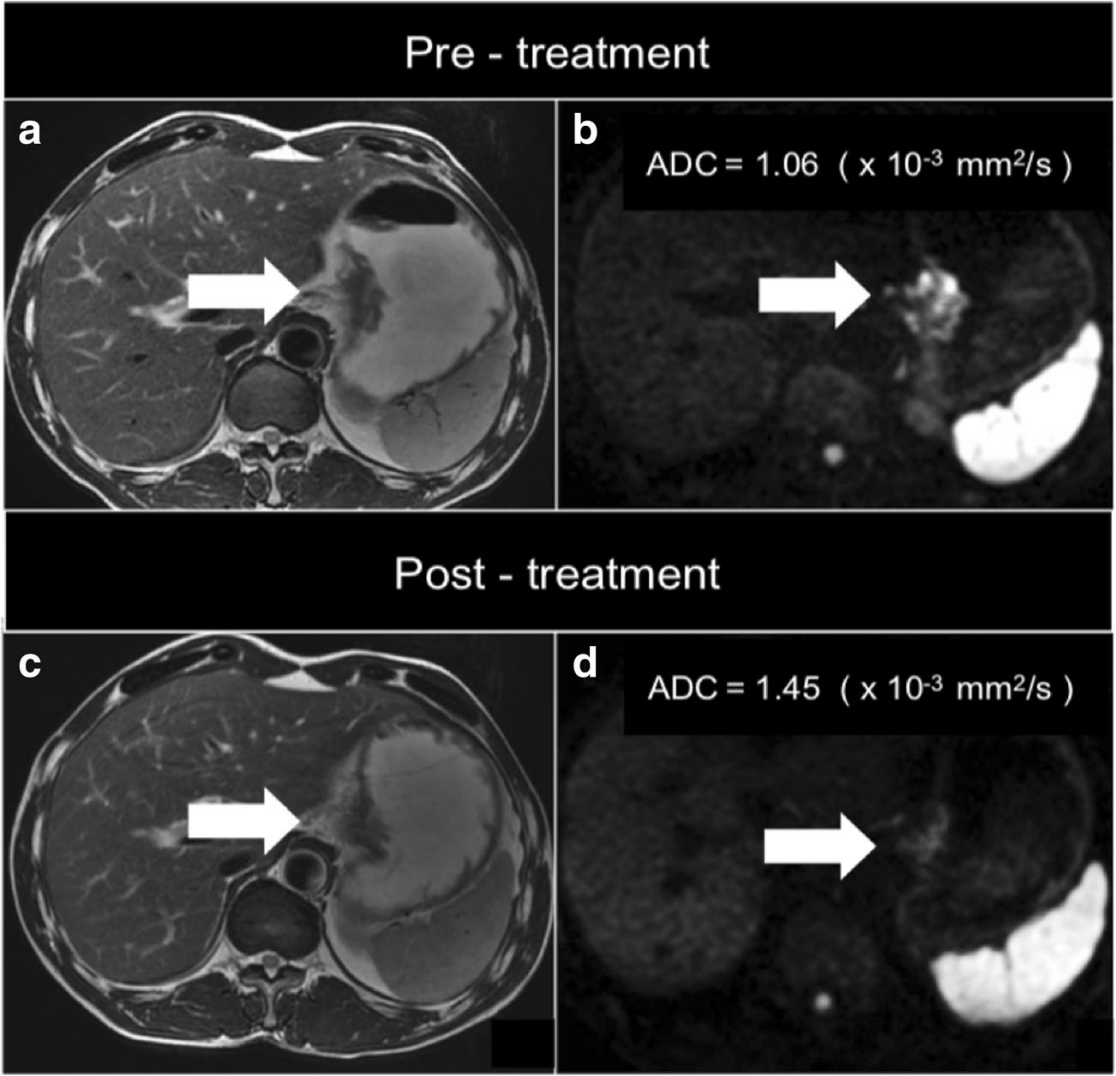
Image of a tumour of the gastro-oesophageal junction on T2-weighted (a and c) and diffusion-weighted (b and d) imaging before and after treatment. There has been a decrease in the conspicuity on DW-MRI (d) and an increase in the apparent diffusion coefficient (ADC) value after treatment (d)
